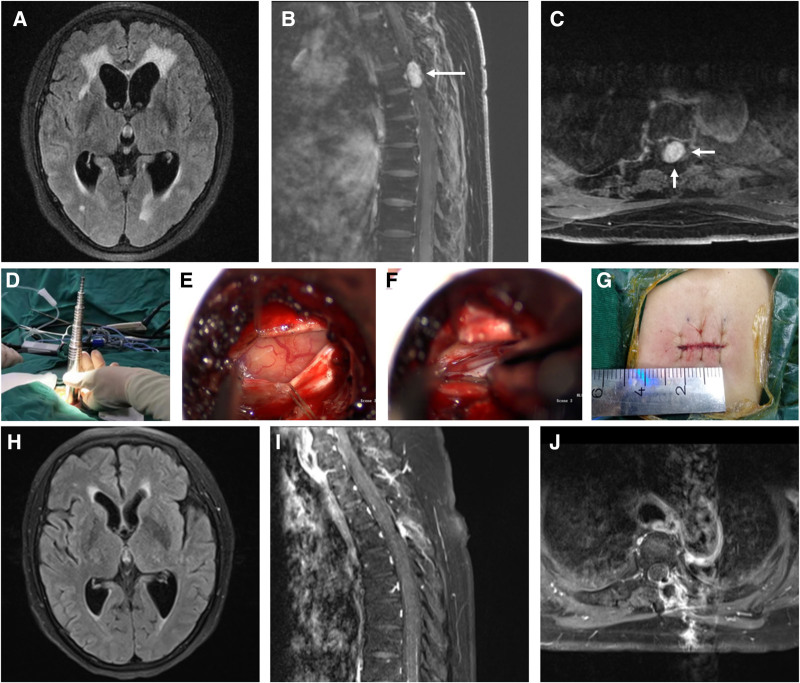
Preoperative, intraoperative, and final follow-up magnetic resonance image in the brain and spine. Preoperative horizontal plane revealed hydrocephalus, axial view of the T2 FLAIR MRI of the head showed ventricular enlargement and periventricular interstitial edema (A). The sagittal and horizontal plane of enhanced magnetic resonance images revealed an ellipsoid mass with well-defined demarcation in the upper thoracic spinal canal (B, C). Intraoperatively established channel system through a 2-cm surgical incision, then the dura mater was incised to reveal the tumor during the surgery (D, E). Intraoperative image showed the complete resection of the tumor (F). A 2-cm surgical incision lateral to the midline at the T4 level is shown (G). At the final follow-up, the MRI scan of the head revealed no recurrence of the tumor and signs of hydrocephalus relieved (H–J). FLAIR, fluid-attenuated inversion recovery; MRI, magnetic resonance imaging.
